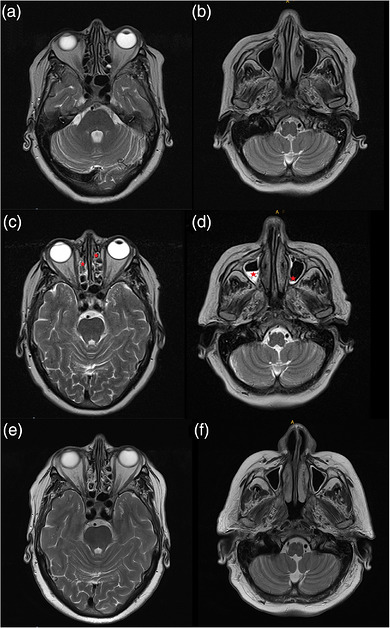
Axial T2‐weighted MRI images of Patient 3 showing (a, b) unremarkable pre‐treatment findings; (c, d) inflammatory changes in the anterior ethmoid cells during ICI therapy (red asterisks); (e, f) near‐complete resolution of mucosal thickening after topical corticosteroid therapy.
